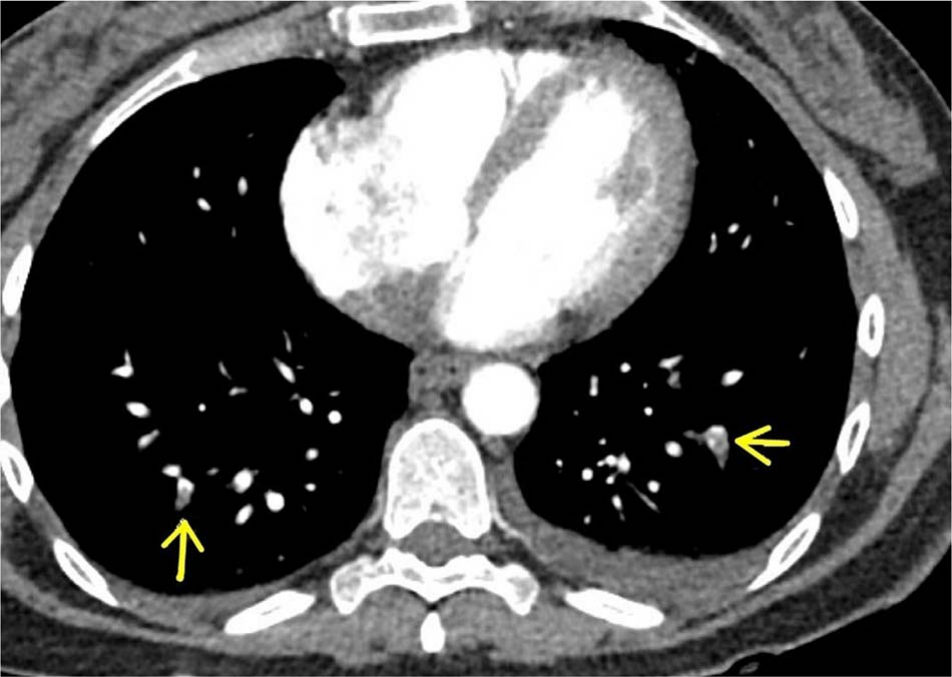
CTA chest (august 2024) showing bilateral subsegmental pulmonary artery embolism (arrows).
